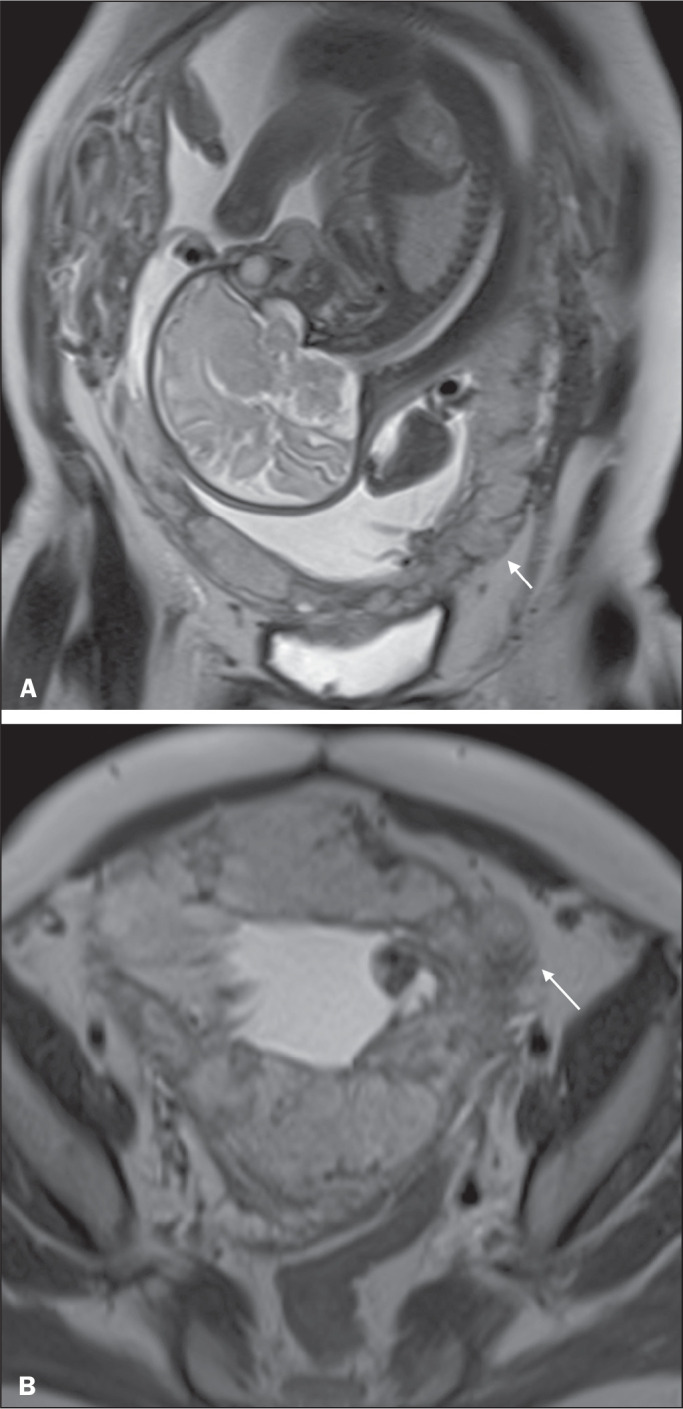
A 35-year-old patient. Coronal T2-weighted HASTE sequence (A) and axial
